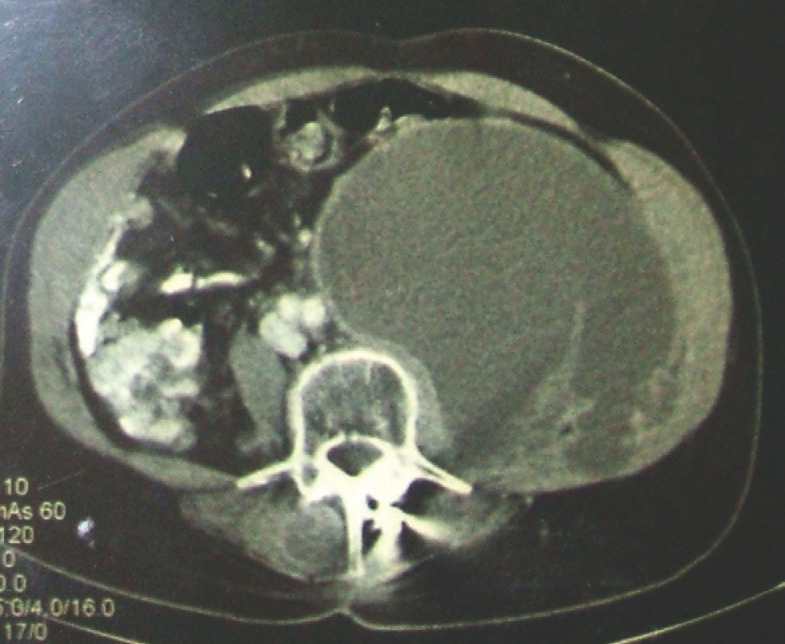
Volumineux abcès initial du psoas gauche vu en tomodensitométrie Voluminous initial left psoas abscess seen on CT scan
